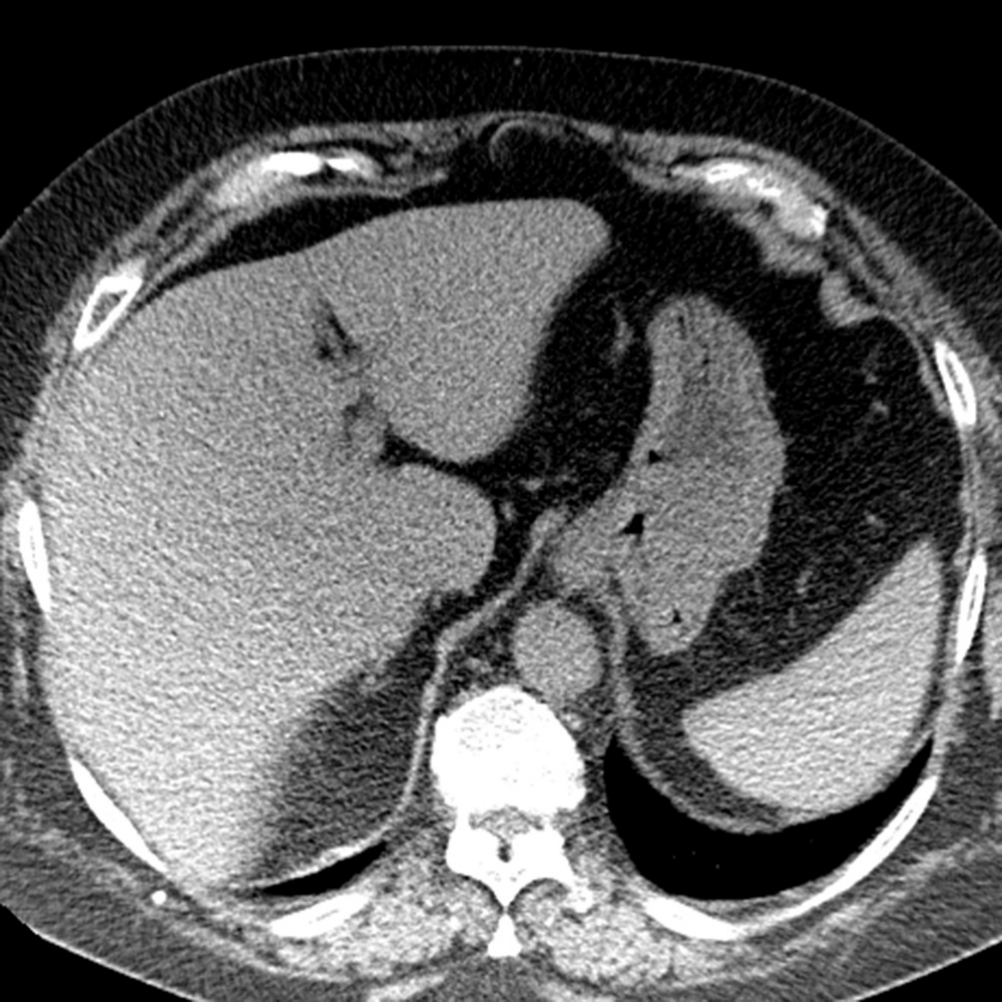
On unenhanced CT scan, the gastric tumour is barely delineated from the surrounding structures and seems to be inaccessible for biopsy through the anterior abdominal wall.
